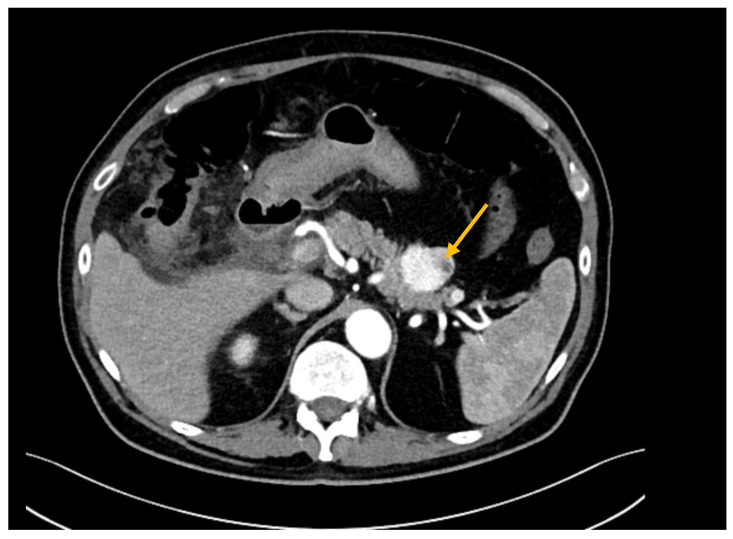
CT scan showing a hypervascular lesion of the pancreatic tail (PM from RCC, yellow arrow).
